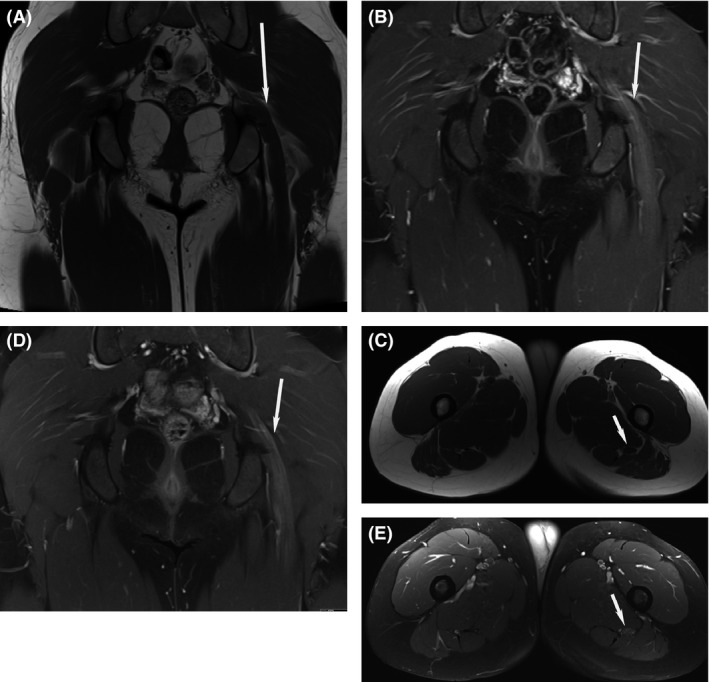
Magnetic resonance imaging (MRI) using coronal T1‐weighted turbo spin echo (TSE) (A), short‐tau inversion recovery (B), and axial T1‐weighted TSE (C) images were obtained. Following gadolinium administration, coronal and axial T1‐weighted fat‐suppressed TSE were obtained (D, E). The perineurioma is marked with arrows.
